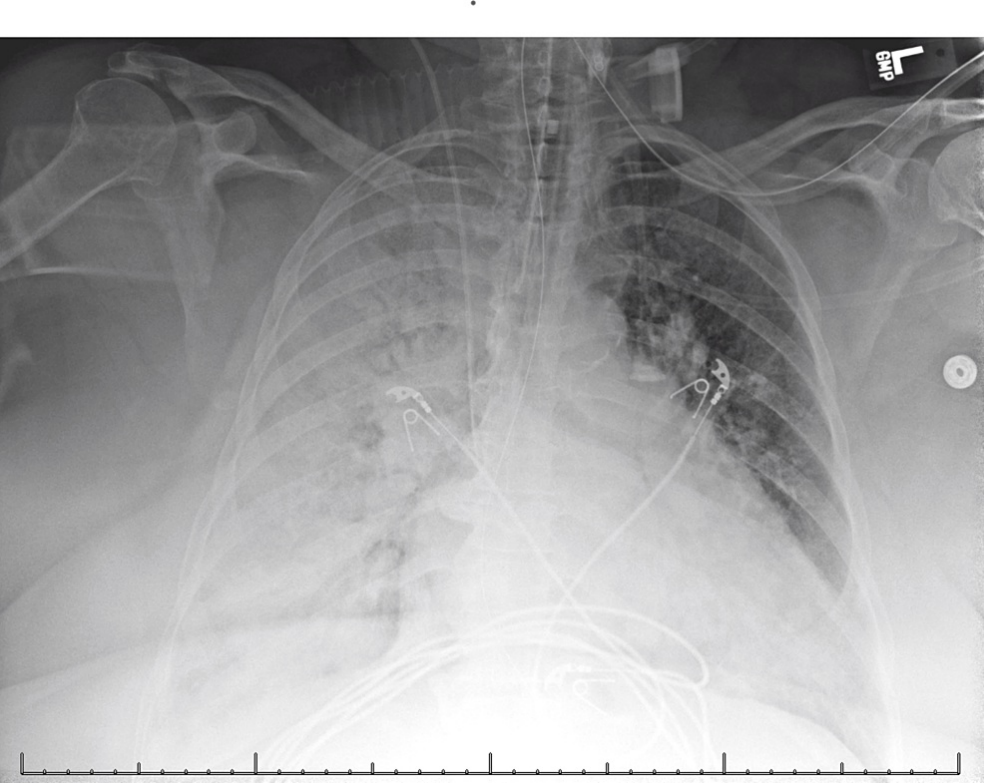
Subsequent chest radiograph showing nearly complete opacification of the right hemithorax and progressive opacification of the left lung base.
